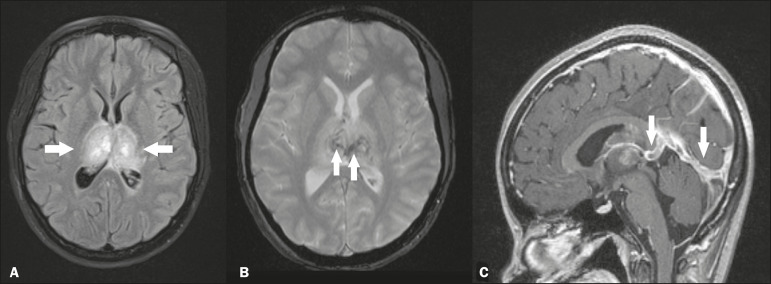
Deep cerebral venous thrombosis in a female patient who used oral contraceptives. A: Axial FLAIR MRI sequence showing hyperintensity and a volume increase in the thalamus (arrows). B: Axial T2*-weighted MRI sequence showing hypointense foci within thalamic lesions (arrows), secondary to hemoglobin degradation products. C: Sagittal-enhanced axial T1-weighted MRI sequence showing a filling defect affecting the straight sinus (arrows). Source: Courtesy of Dr. Márcio Tadeu Vieira de Brito.
